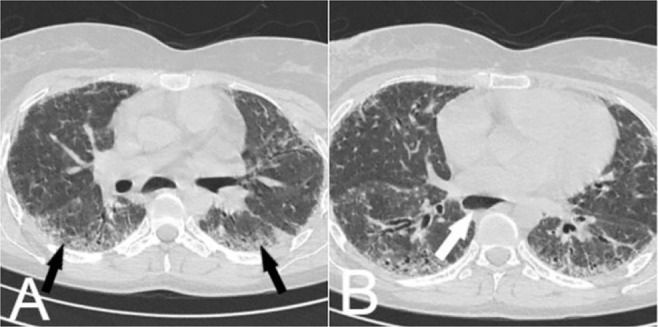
A)Non-contrast axial chest CT scan of 54-year-old woman known case of scleroderma showed sub-pleural GGO, reticulation, bronchiectasis and dilated esophagus (white arrow in B) that are typical for NSIP.
